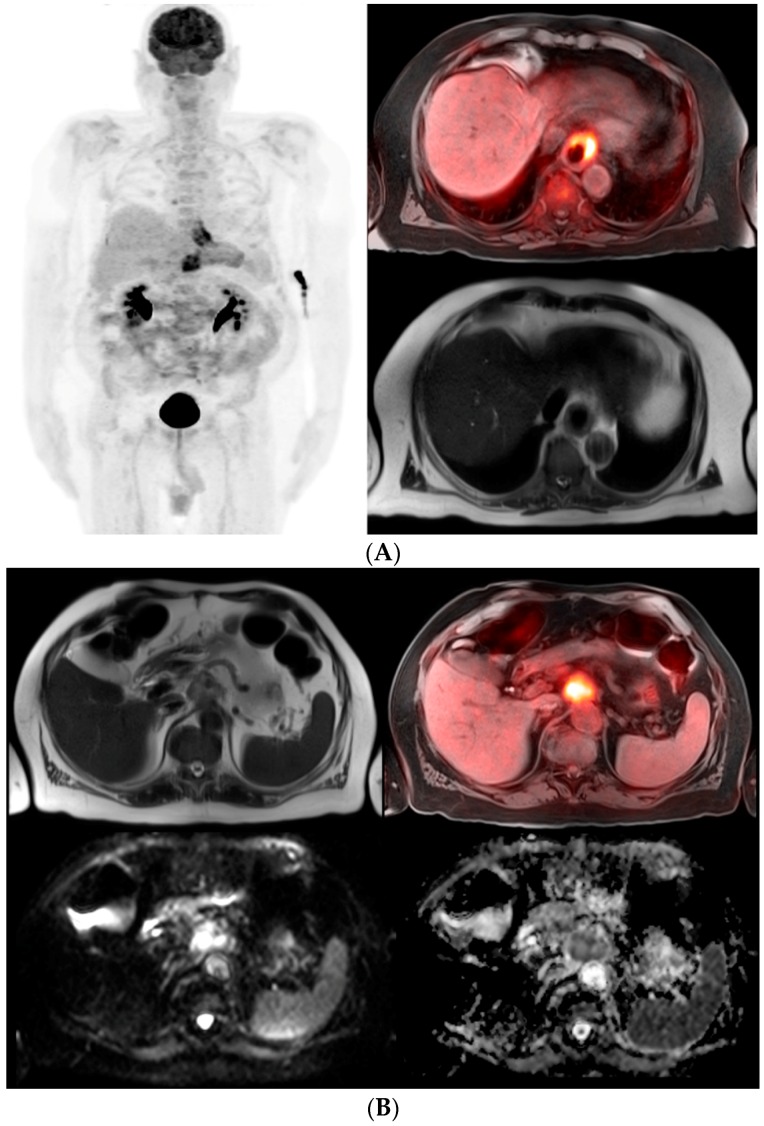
(A) 71-year-old male with a history of esophageal adenocarcinoma with an esophageal stent. PET maximum intensity projection (MIP) images demonstrate two abnormal FDG foci corresponding to the distal esophageal mass and an upper abdominal metastatic lesion (*left image*). The fusion PET-MRI transaxial image using T1 with fat suppression shows prominent abnormal FDG uptake in the distal esophageal cancer (*top right*). The T2 haste transaxial image demonstrates eccentric esophageal wall thickening (*bottom right*); (B) Metastatic lymph node from esophageal adenocarcinoma. The T2 haste transaxial image shows an enlarged lymph node located superior to the celiac axis of the aorta (*top left*). Fusion PET-MRI image using T1 with fat suppression reveals prominent abnormal FDG activity consistent with metastasis (*top right*). Diffusion weighted images demonstrate hyperintense signal in the enlarged lymph node (*bottom left*) with corresponding low signal on the apparent diffusion coefficient map (*bottom right*) which is consistent with malignancy.
